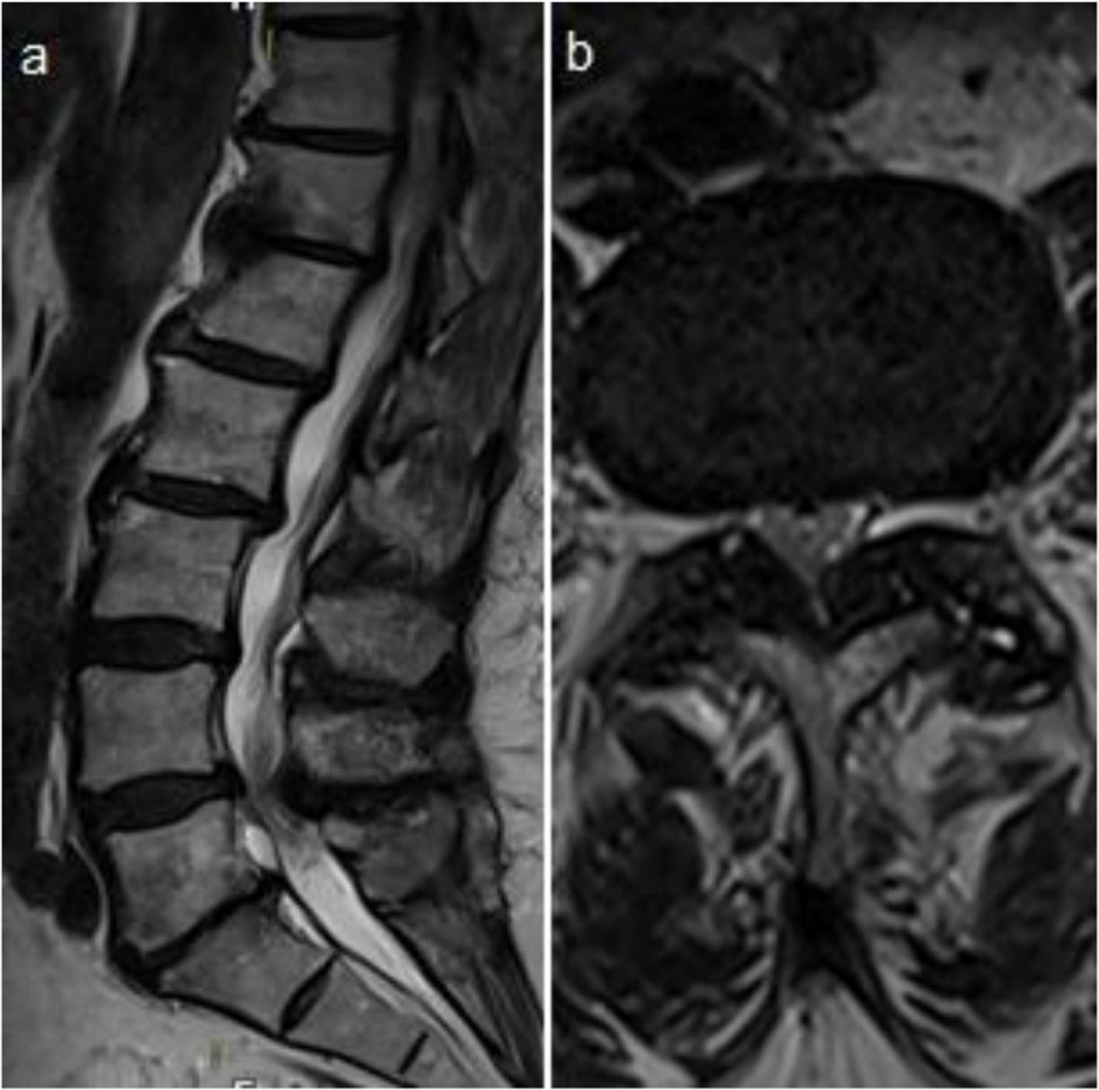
Preoperative sagittal (a) and axial (b) T2-weighted MRI showing a spinal stenosis at the level of L4/5. Note, that there is no facet gap joint preoperatively
